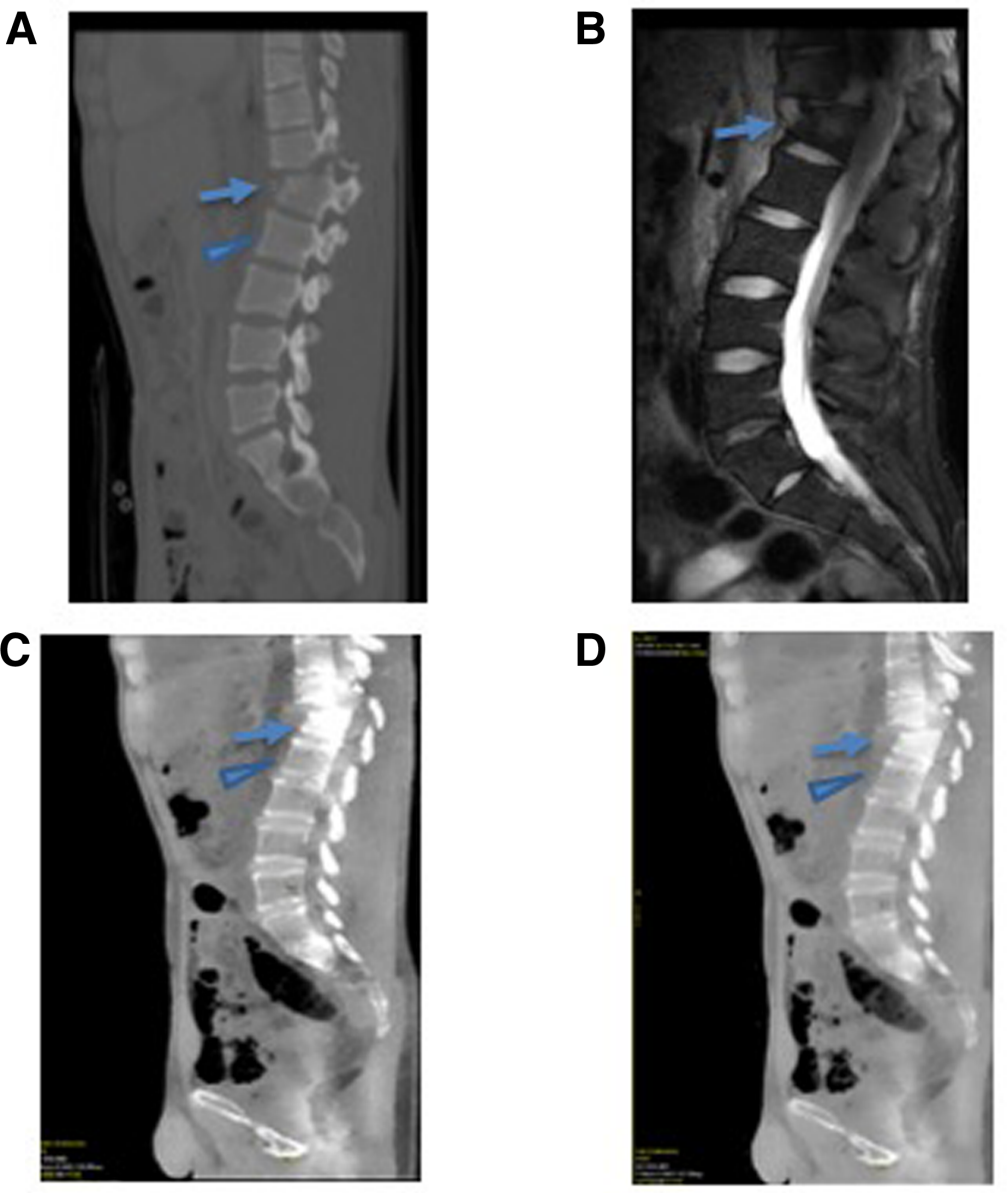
A 25-year-old male presenting with an acute thoracic spine fracture after a fall from a tree based on GSI dual-energy CT analysis with MRI correlate. (A) The conventional CT scan of the thoracic spine demonstrates a fractured T12 (arrow) and uninvolved L1 vertebral body (arrowhead). (B) The MRI STIR imaging demonstrates an increased signal within the fractured vertebral body (arrow) without a signal abnormality in the uninvolved adjacent L1 vertebral body (arrowhead). (C) Water (calcium) and (D) fat (calcium) images demonstrated a quantifiably increase in water content at the fractured T12 vertebral body (arrow) compared with the normal L1 vertebral body (arrowhead).
